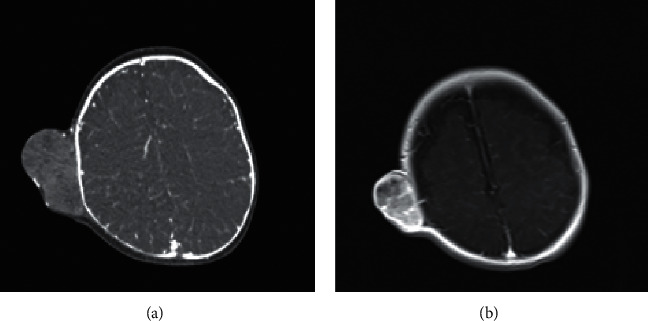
Contrast-enhanced CT demonstrating right parietal vividly enhancing scalp lesion without involvement of the skull (a). Contrast-enhanced MRI also showing vivid enhancement (b).
